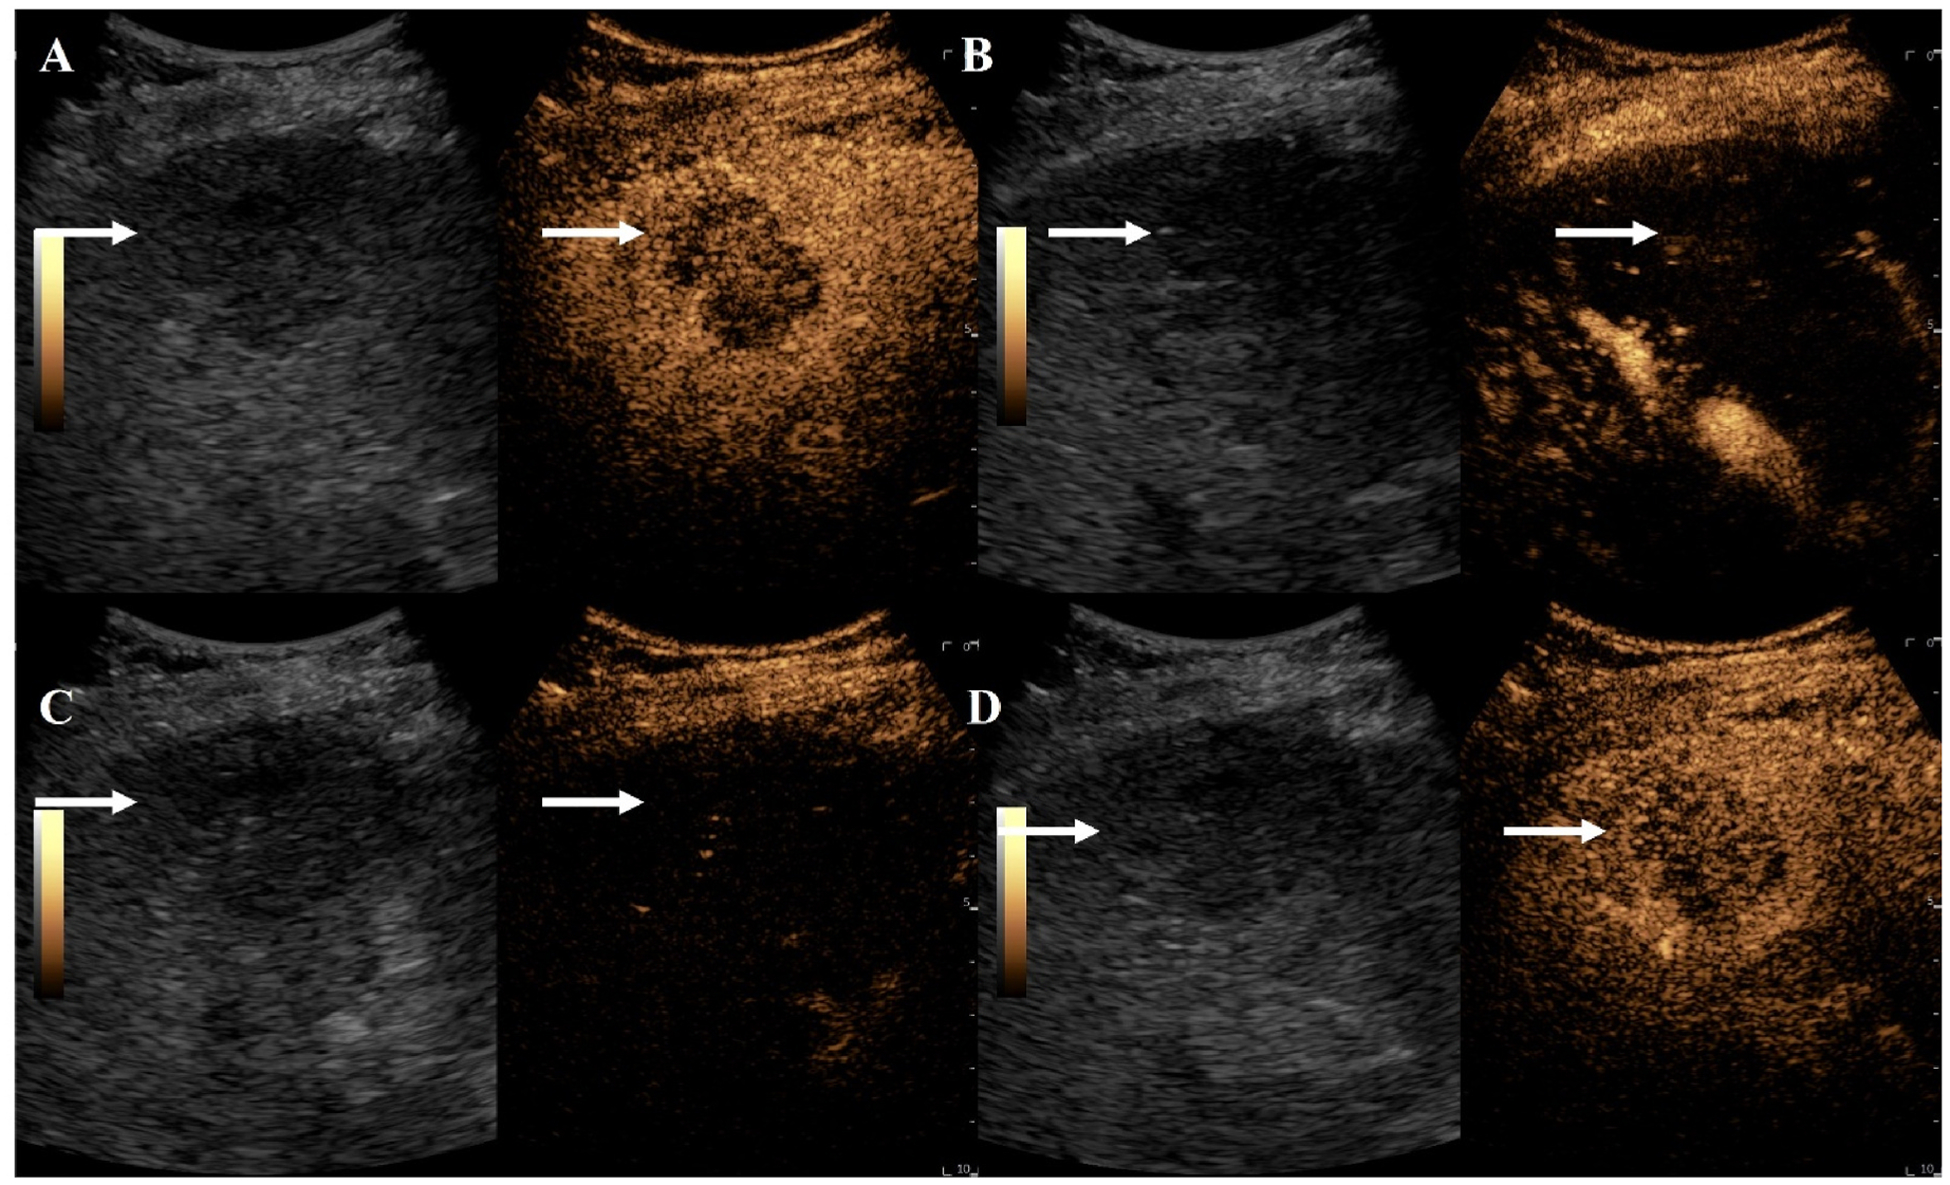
An example of 2D CEUS UTMD imaging. 2D UTMD CEUS imaging of an intrahepatic cholangiocarcinoma participant with a 6.1 cm segment 5/6 tumor 2 weeks post Y90-TARE. (A) Dual non-linear harmonic imaging (right) and B-mode imaging (left) at peak enhancement demonstrating viability within the tumor. (B) An UTMD with high intensity ultrasound pulse applied to the tumor (ISPTA = 165.9). (C) Immediately post UTMD demonstrating almost complete destruction of ultrasound contrast agents within the field of view and (D) reperfusion of ultrasound contrast agents in the tumor demonstrating continued enhancement at 2 weeks post-treatment correlating with stable disease on mRECIST. White arrow indicates the intrahepatic cholangiocarcinoma. UTMD = ultrasound-triggered microbubble destruction, CEUS = contrast-enhanced ultrasound, Y90-TARE = yttrium-90 transarterial radioembolization, ISPTA = intensity spatial peak temporal average, mRECIST = modified response evaluation criteria in solid tumors.
